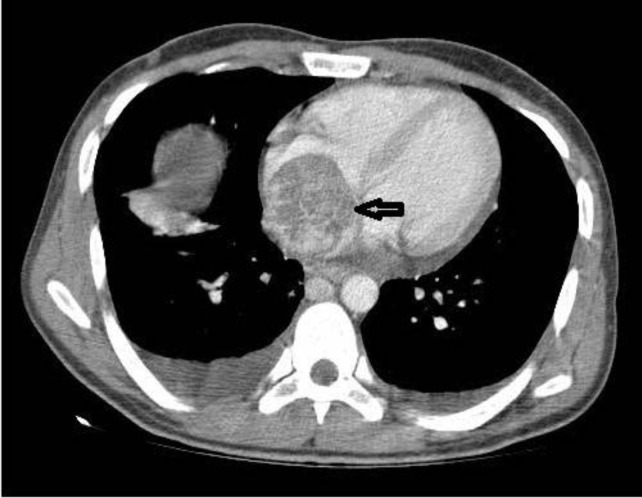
Contrast-enhanced chest computed tomography revealed large heterogeneous mass in right atrium (black arrow) and bilateral pleural effusion.
